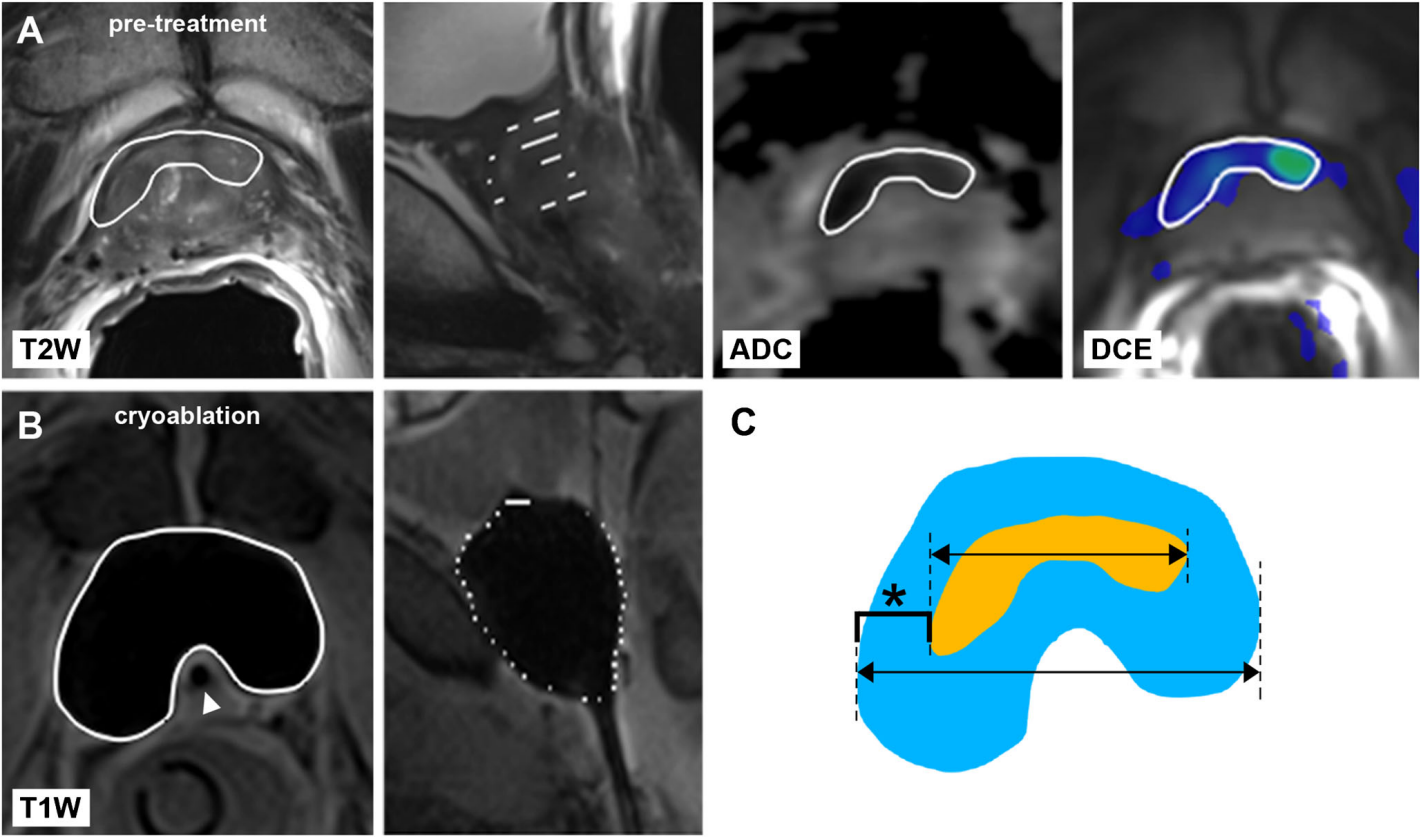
Annotation of a tumour (*outlined in white*) on pretreatment axial and sagittal T2-weighted, ADC and DCE prostate MR images and b corresponding iceball (*outlined in white*) at the end of the second freeze cycle on axial and sagittal intraprocedural T1-weighted images, with urethral warming catheter in situ (*arrowhead*). c The iceball margin (*asterisk*) was determined by subtracting the radii of the tumour (*yellow*) and iceball (*blue*) along each direction
